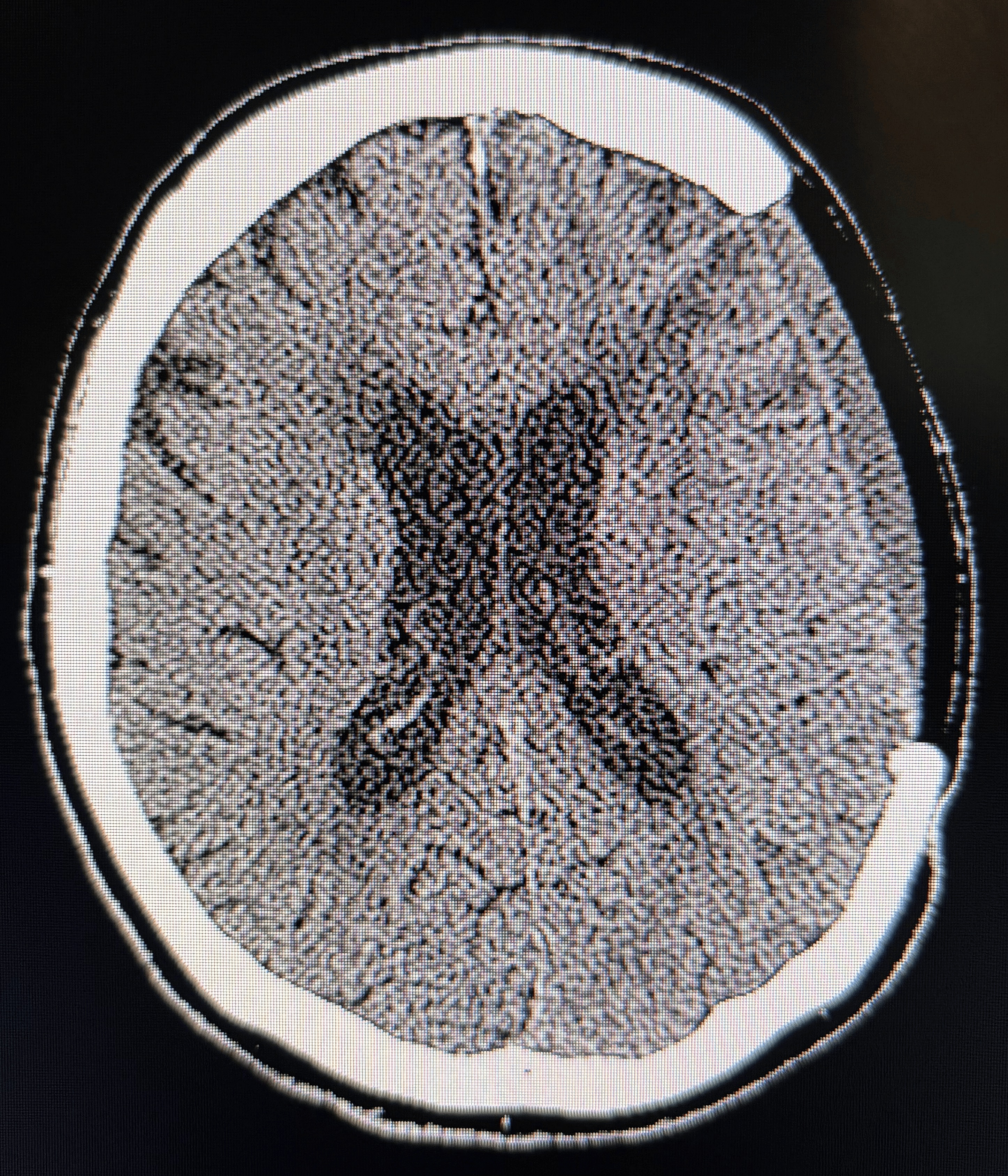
Long-term Resolution 5 months post-cranioplasty, CT imaging shows significant improvement in the patient’s mass effect.
